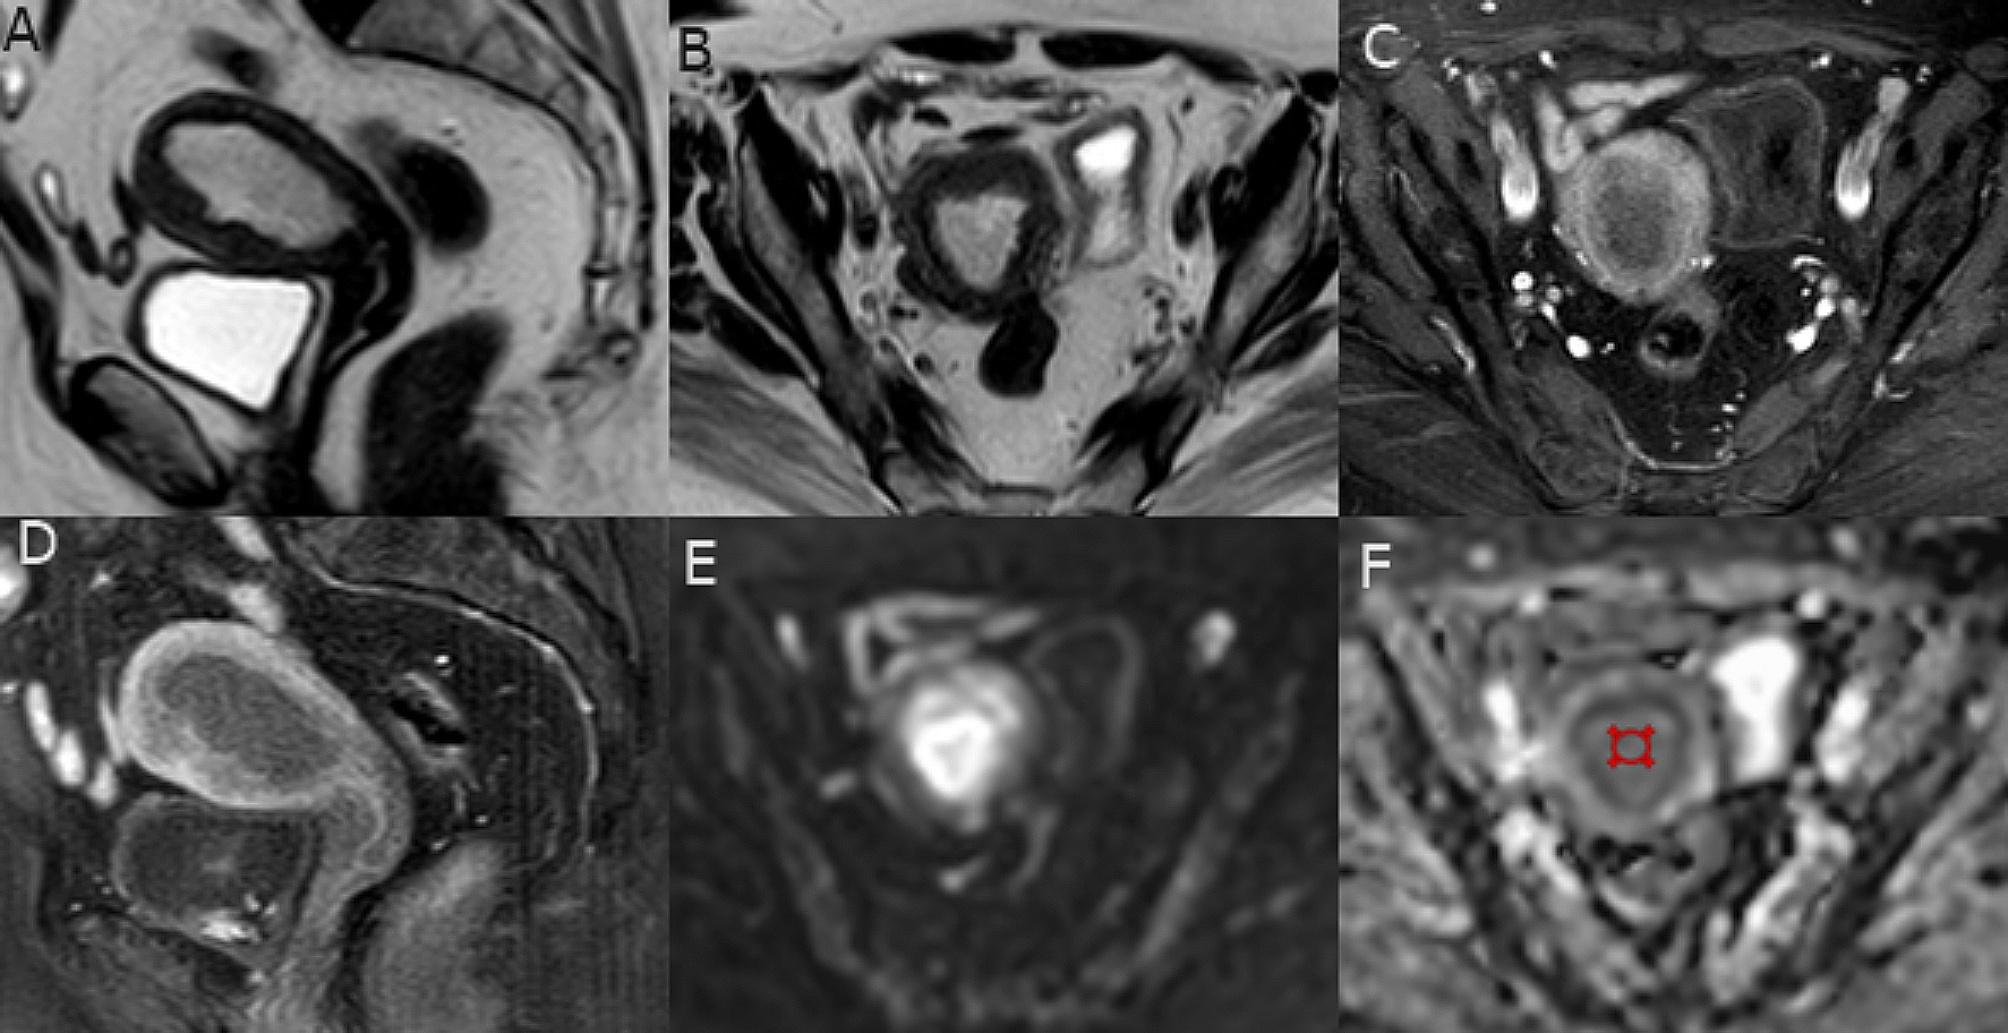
A patient with grade 2 endometroid carcinoma, no LVSI (FIGO stage IA). (A and B) Sagittal and axial oblique T2-WI respectively showed the endometrial mass of intermediate to high signal intensity with focally interrupted hypointense junctional zone denoting superficial MI, no detected CSI nor pathological pelvic LNs. (C & D) Axial oblique and sagittal contrast-enhanced T1-WI revealed interrupted sub-endometrial enhancement with hypo-enhancement of the endometrial mass. (E & F) Axial DWI at b = 1000 and ADC map respectively revealed diffusion restriction of the endometrial mass with ADCmean value = 1.02 × 10 –3 mm/sec
